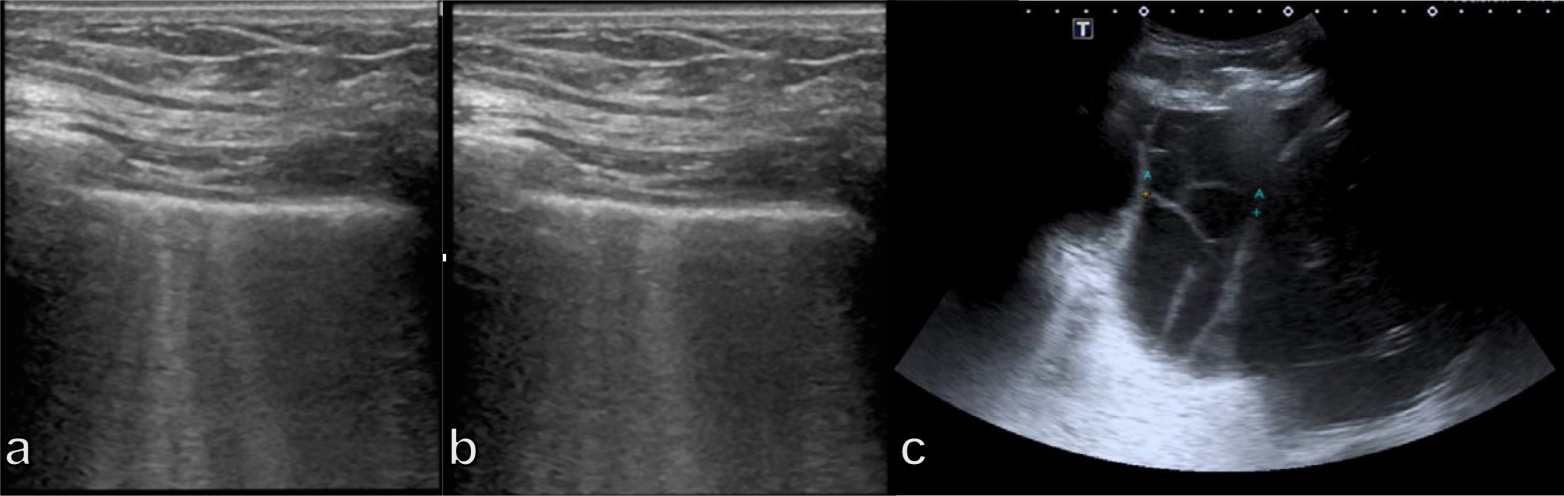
(a, b) Lung ultrasound of a 61-year-old male dyspneic patient depicting B-lines indicative of cardiogenic pulmonary edema; (c) 17-old-year male patient with complex parapneumonic pleural effusion showing septa.
